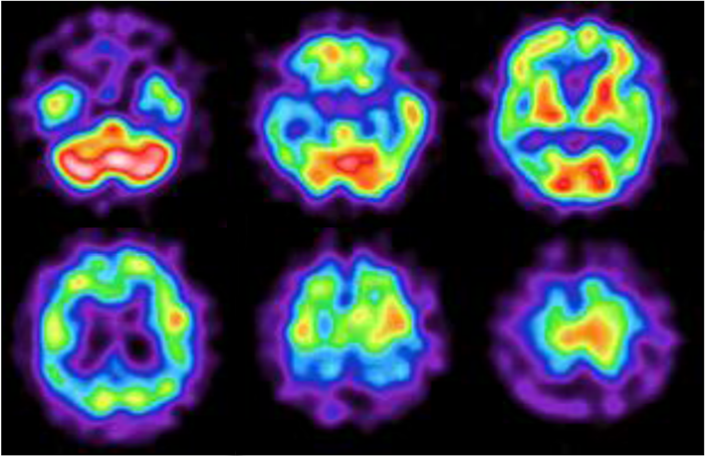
I-IMP SPECT of the proband (III-10) at the age of 53. A generalized decrease drop in cerebral blood flow is shown.
